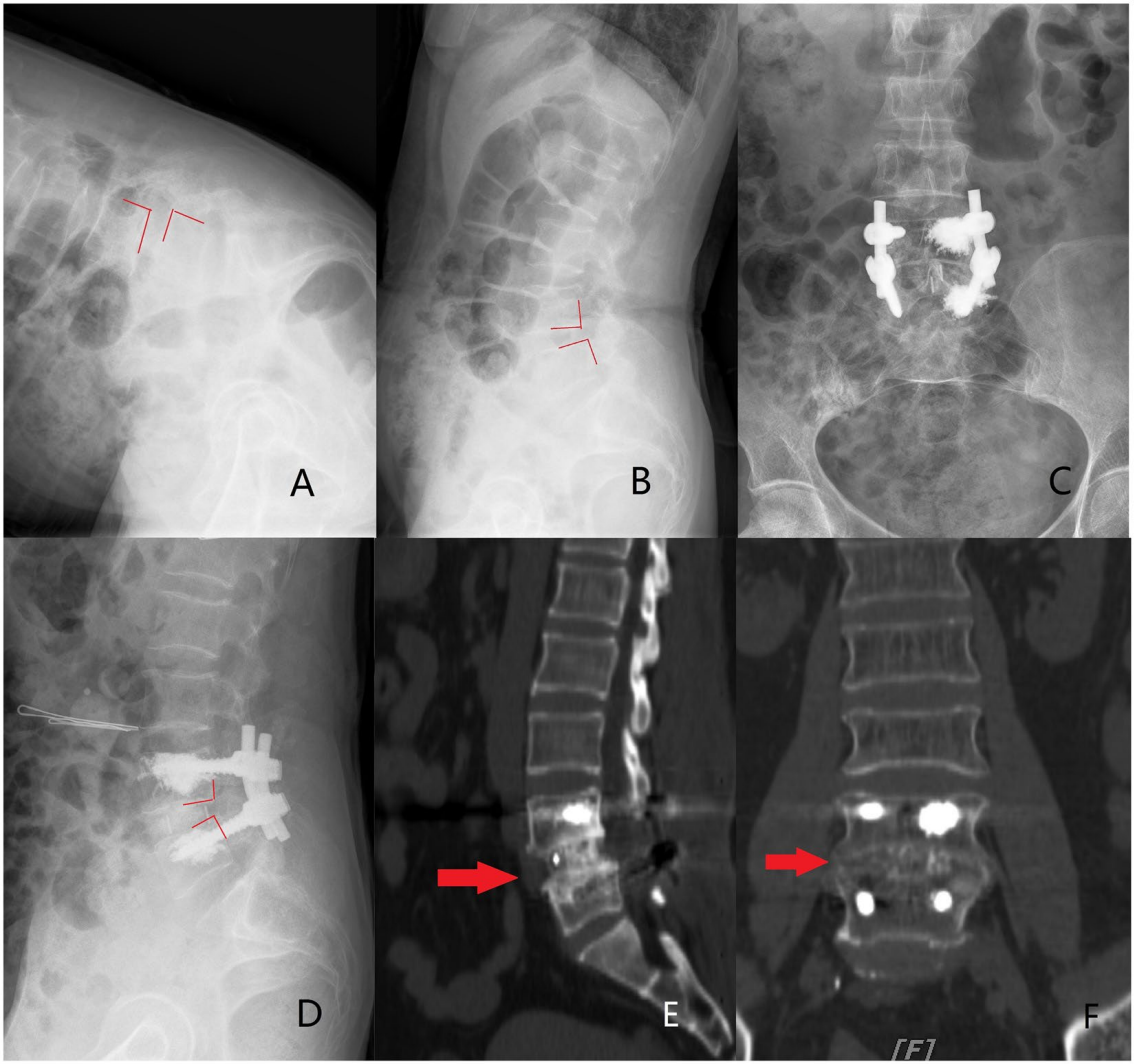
A 69-year-old female (T = −3.6) who was treated with unilateral 2 CICPSs fixation by PMMA augmentation. (A,B) Preoperative extension and flexion radiographs indicated the L4 vertebral body was unstable and displaced forward. PMMA seemed to leak to the anterior vertebral body. (C,D) The postoperative radiographs showed lumbar sequence reconstruction. (E,F) Three years after surgery, CT showed bony fusion achieved (the red arrow).
